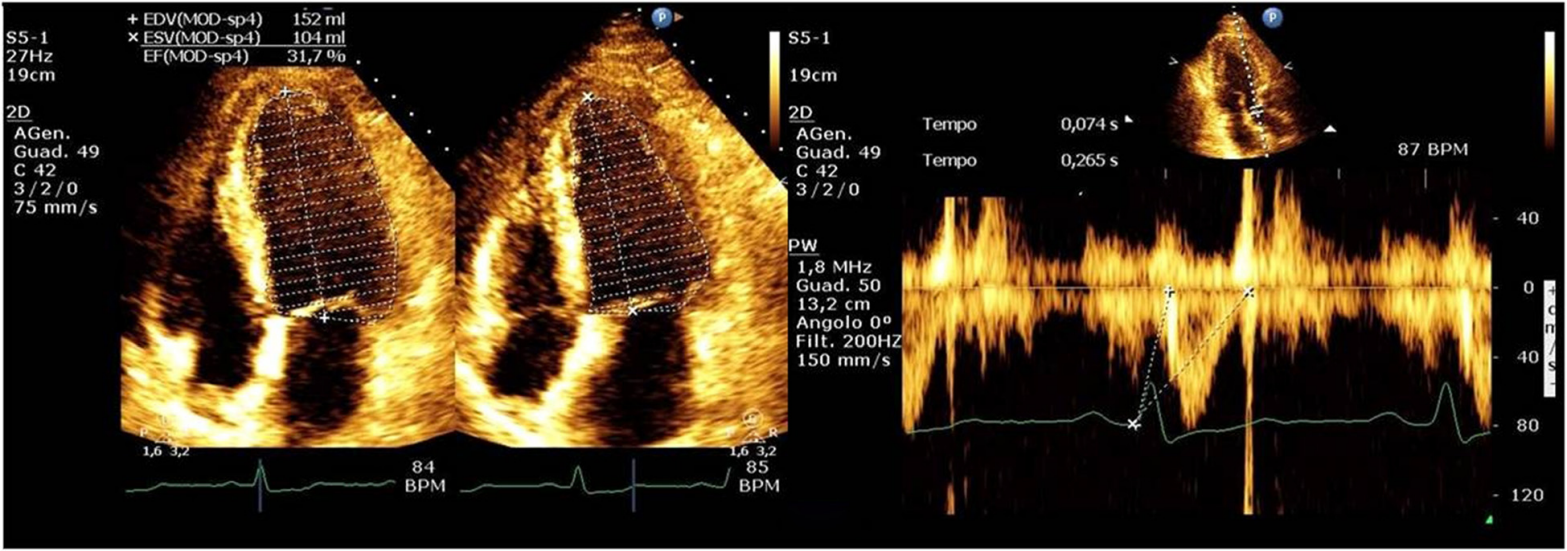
Left ventricular end-systolic elastance was calculated by the single-beat method validated by Chen et al. [14]. The echo figures display the evaluation of ejection fraction (left panel) and pre-ejection and ejection time (right panel) using aortic Doppler waveform. Normalized ventricular elastance at arterial end-diastole (End) was measured according to the formula: *E*_*es*(*sb*)_ = [*P*_*d*_ - (*E*_*Nd*(*est*)_ • *P*_*s*_ • 0.9)]/[*SV* • *E*_*Nd*(*est*)_], *E*_*Nd*(*avg*)_ = ∑ *ai* • *t*_*Nd*_ *i* = 0, *E*_*Nd*(*est*)_ = 0.0275 - 0.165 • *EF* + 0.3656 • (*P*_*d*_/*P*_*es*_) + 0.515 • *E*_*Nd*(*avg*)_ where a_i_ are (0.35695, -7.2266, 74.249, -307.39, 684.54. -856.92, 571.95, -159.1) for i = 1 to 7, respectively. The value for t_Nd_ was determined by the ratio of pre-ejection period (R wave to flow onset) to total systolic period (R wave to end-flow), with the time of onset and termination of flow defined Doppler. Systolic (Ps) and diastolic (Pd) blood pressure were invasively obtained.
